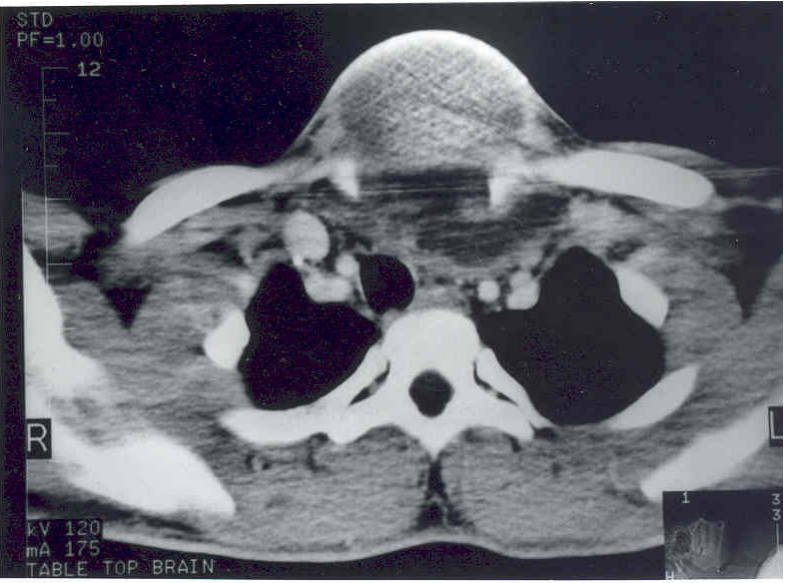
Contrast-enhanced CT scan of the upper thorax showing extension of a complex cystic mass and displacement of the trachea with compression of major vessels.
